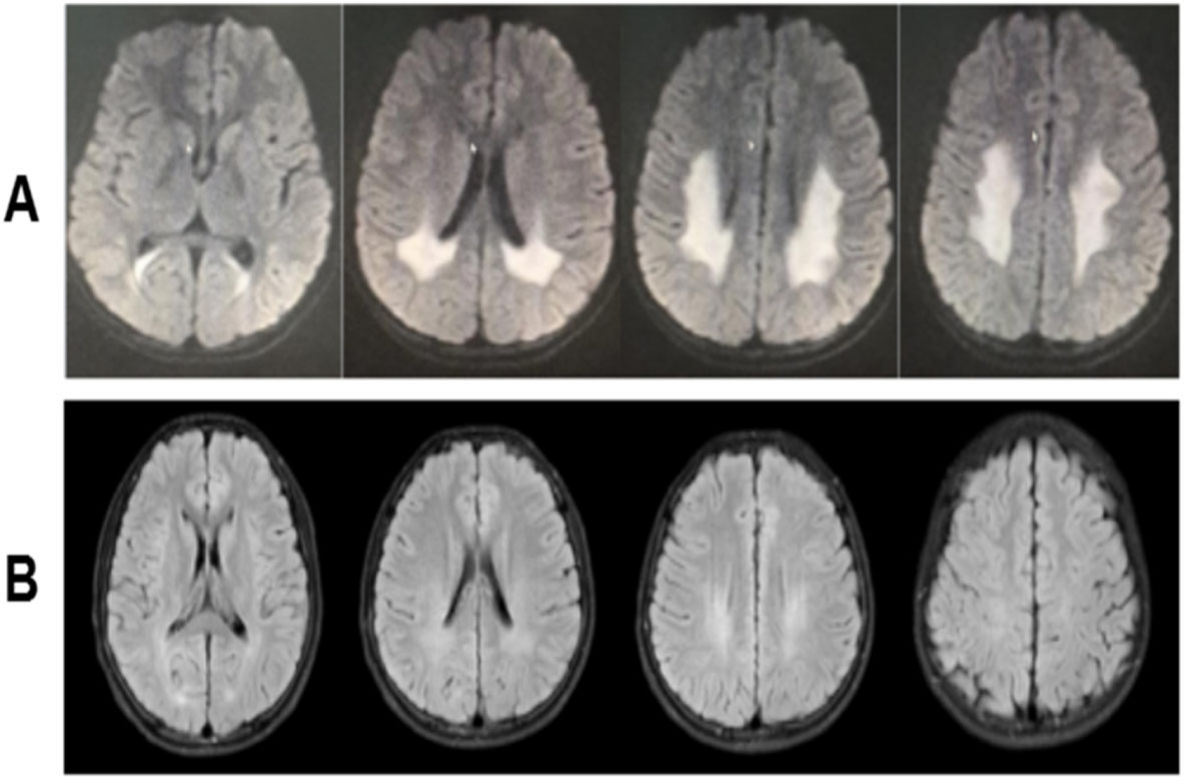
Brain MRI of patient 2. a: MRI on 17 February 2018 indicated white matter lesions in bilateral posterior ventricular areas; b: White matter lesions nearly complete resolution on 6 March 2018. MRS was normal
